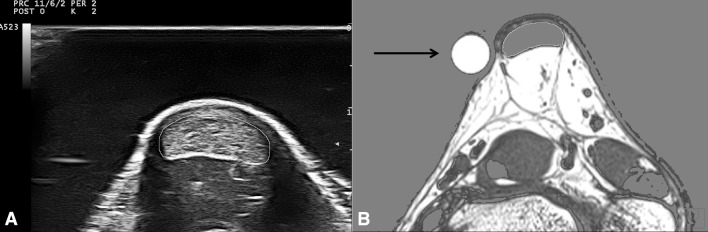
a Transversal US image demonstrating the manually outlined Achilles tendon CSA (*yellow* shape) and b transversal T1-weighted MRI image showing the automatically outlined Achilles tendon CSA (*yellow* shape) with the spherical marker (*black arrow*) to the left (color figure online)
